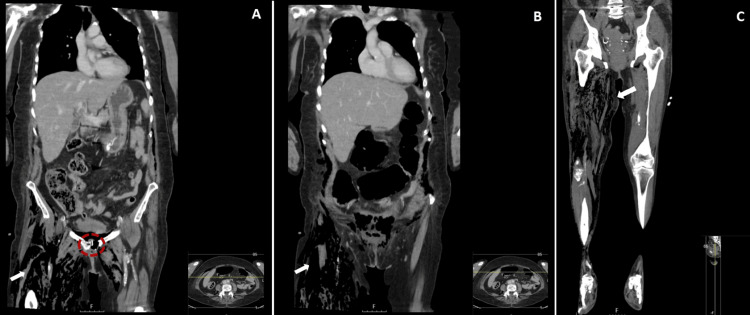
Coronal CT images of the abdomen, pelvis, and lower limbs (A–C) Sequential coronal CT images showing subcutaneous and intermuscular emphysema extending from the anterior abdominopelvic wall to the thighs (white arrows). Cortical discontinuity and osseous pneumatosis of the pubic symphysis are also observed (red dashed circle). These findings are consistent with NF and associated osteomyelitis.
